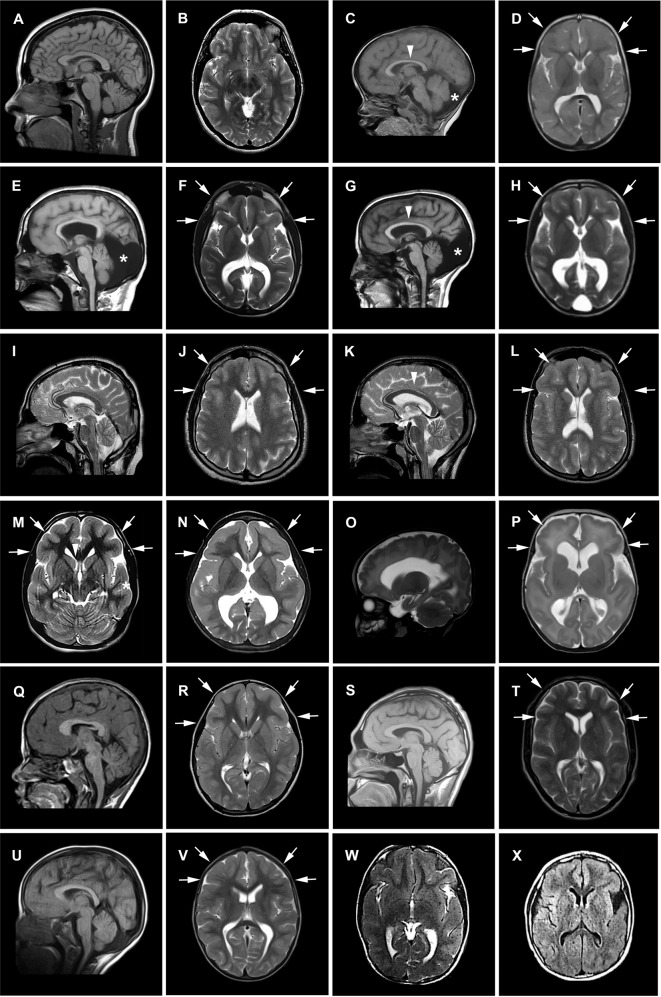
Neuroradiological features of patients with PIDD1 variants. Representative mid-sagittal and axial brain MR images are shown. A, B Mid-sagittal and axial images of an unaffected individual (normal). C, D Images of subject IV:3 of family 1 showing anterior-predominant pachygyria (arrows), thin corpus callosum (arrowhead), mild cerebellar vermian hypoplasia with a retrocerebellar cyst (asterisk). Images of family 2 (E, F G-IV:3; G, H IV:4) showing severe anterior-predominant undersulcation of the cortical gyral pattern consistent with pachygyria and foreshortening of the frontal lobes (arrows), thin corpus callosum, and retrocerebellar cyst (or mega-cisterna magna) (asterisk). Images of family 3 (I, J IV:1; K, L IV:3) showing frontal-predominant undersulcation of the cortical gyral pattern, with no cerebellar or callosal abnormalities (arrows). Axial images of family 4 (M IV:2; N IV:3) showing frontal-predominant pachygyria with foreshortening of the frontal lobes (arrows). IV:3 also has ventriculomegaly (limited views). O, P Images of subject IV:1 of family 5 showing severe undersulcation and pachygyria diffuse (more pronounced frontally; arrows) with ventriculomegaly. Q, R Images of family Manipal-1 (IV:3) (previously described) showing anterior-predominant pachygyria (arrows) and thick genu of the corpus callosum (arrowhead). S, T Images of affected subjects of families AS110 V-3 (previously described) showing undersulcation of the frontal, temporal and parietal regions resembling lissencephaly (arrows). U, V AS105 III-5 (previously described) showing frontal pachygyria with mildly to moderately thick cortex and mild hypoplasia of the corpus callosum. W, X axial images of family 6 showing diffuse undersulcation of the cortical gyral pattern with a thick appearing cortex on limited views.
